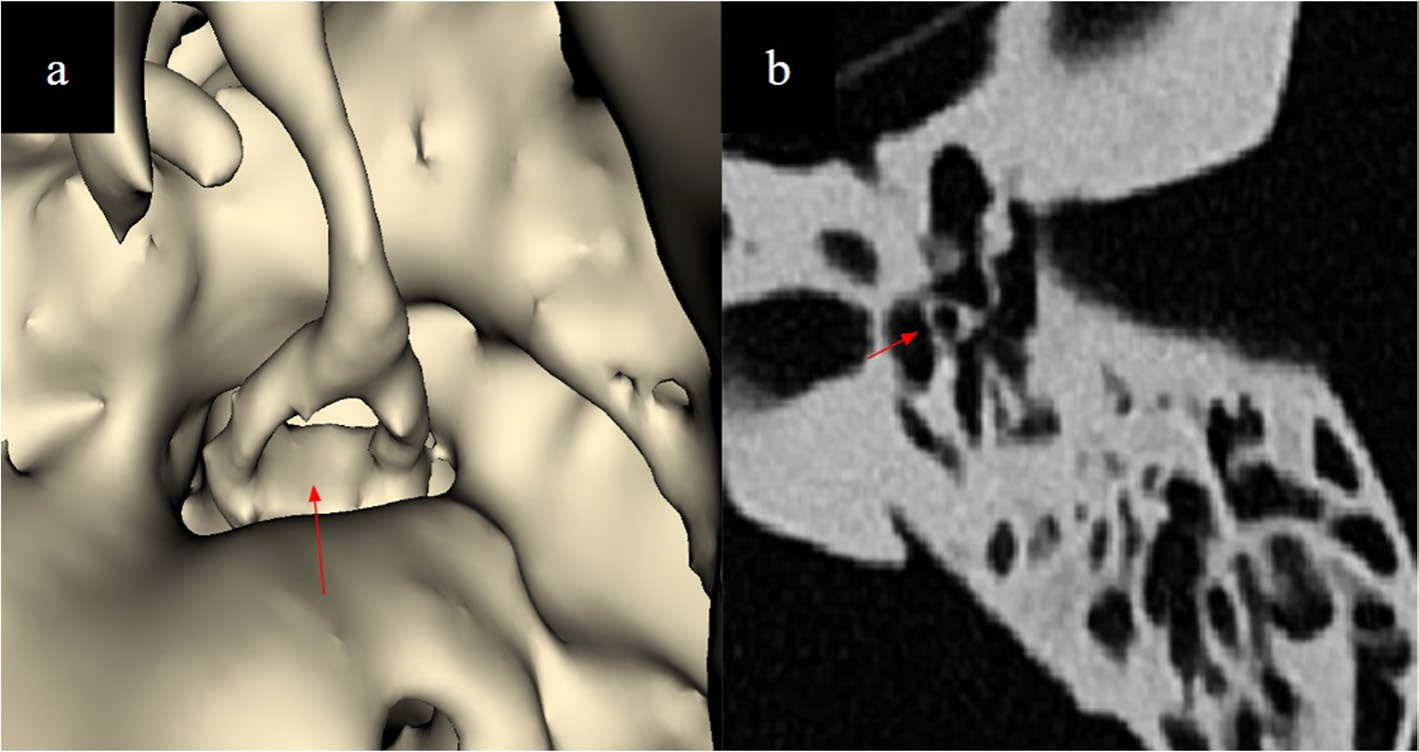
a CAD modelling of the stapes footplate located perpendicular to the opening of the oval window and both crus of the stapes (b) imaging correlate on CT scan of the model. This is not well visualised on CT despite HU thresholding due to its thin diameter and low density
